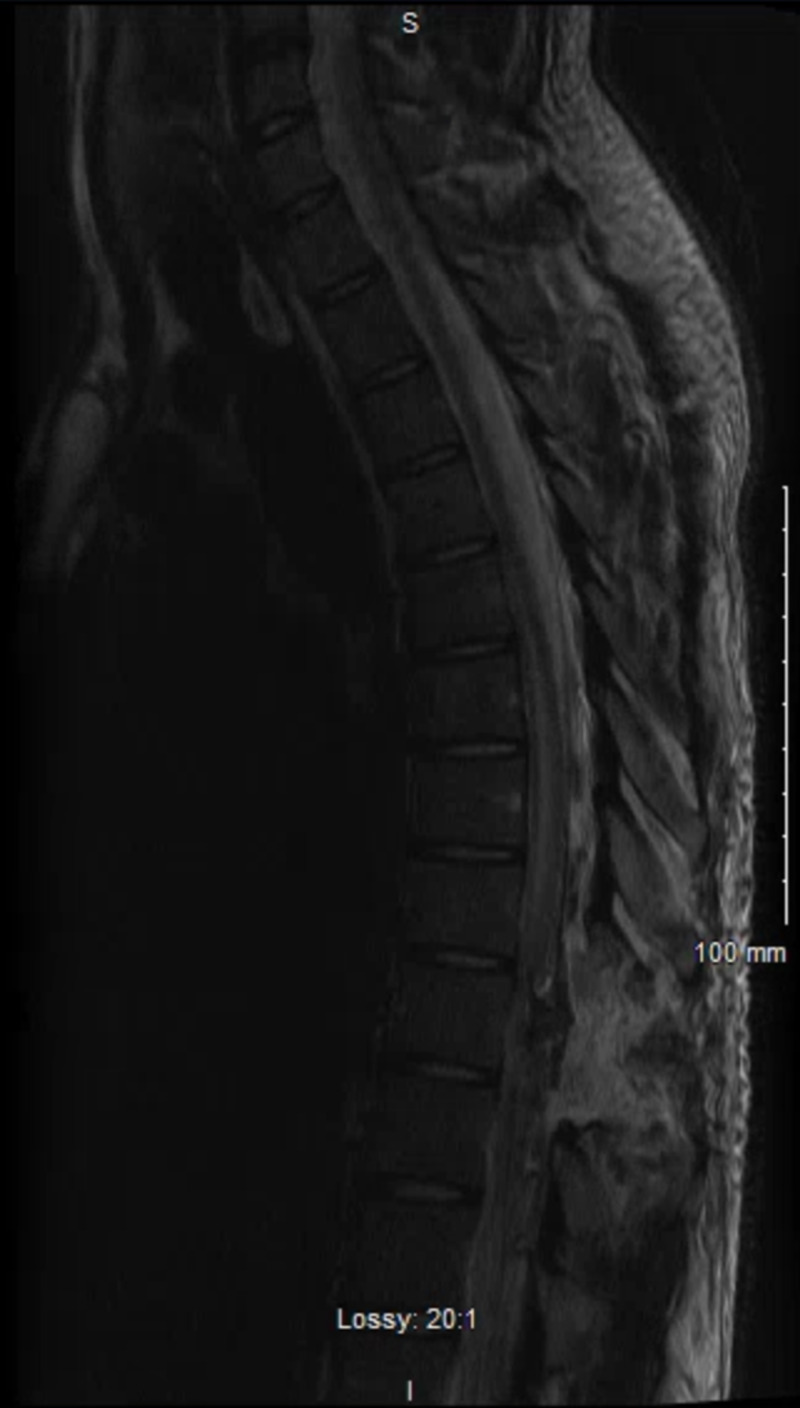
Postoperative MRI with and without contrast, T2 weighted.
